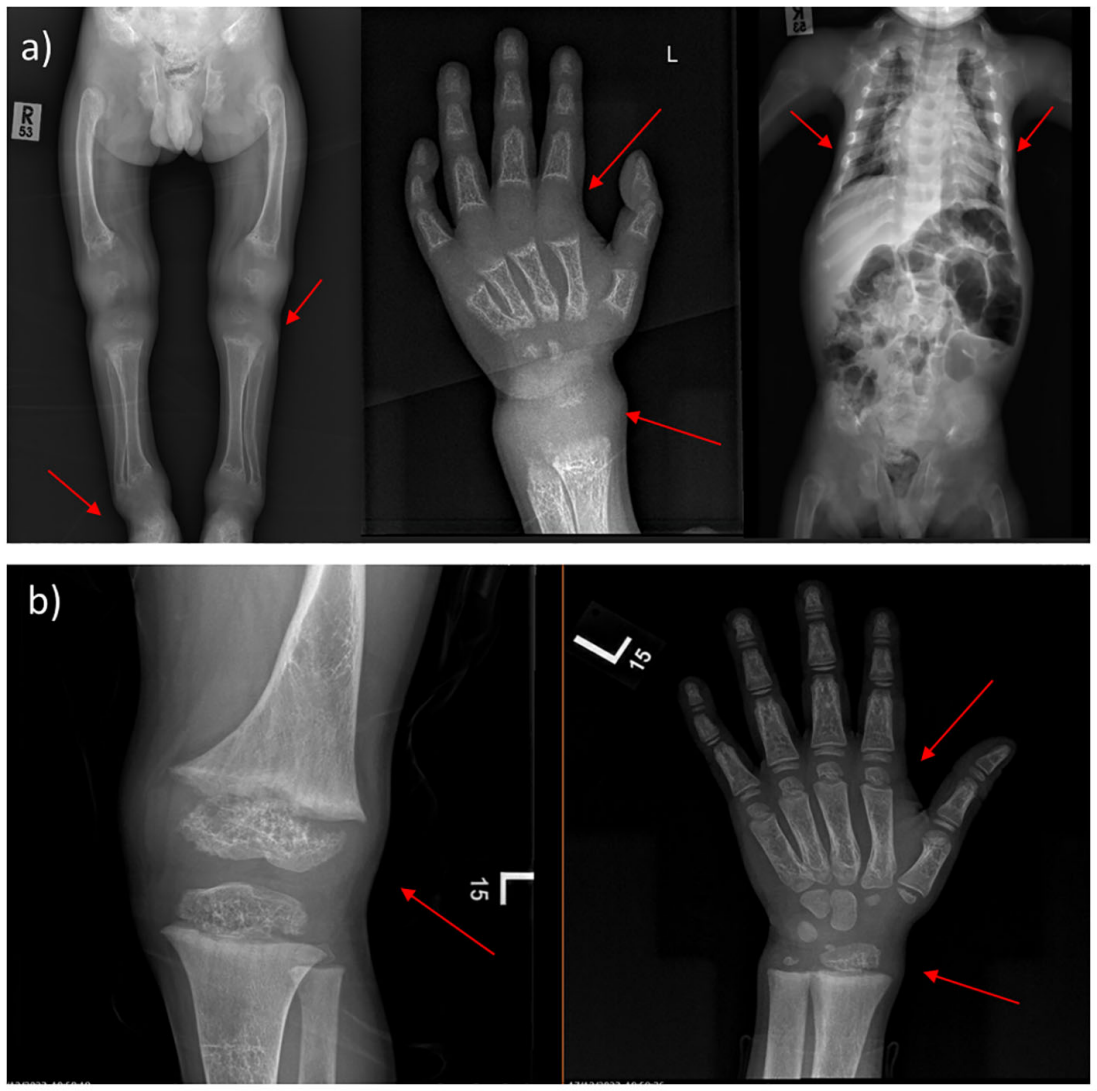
X-ray findings of 4-year-old male. (A) Before treatment, the images showing generalized decreased bone density, the profound widening of the growth plate with metaphyseal cupping and fraying of bilateral hips, ankles, knees, and wrists. Narrow chest wall with evidence of significant ricketic changes and costochondral junction fraying. (B) One year and a half after treatment (6 weeks IV calcium, then maintained on oral calcium 5 gm every 6 hours daily); showing interval improvement compared to the previously seen growth plate widening and metaphyseal irregularity/fraying, with interval metaphysical sclerosis in keeping with healing rickets. (Case 2 in the Supplementary File).
